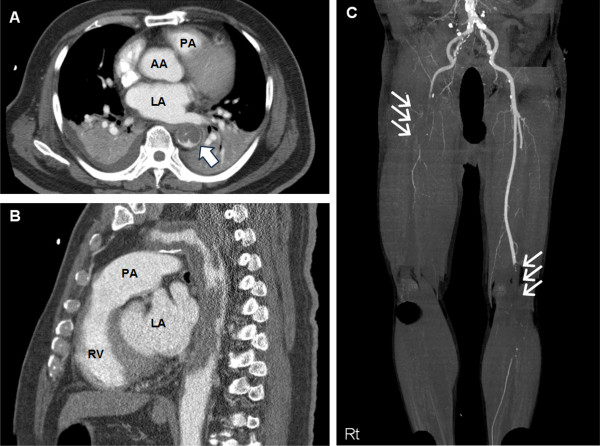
Images of the patient’s 3DCT before surgery. (A) Axial and (B) sagittal section 3DCT scans of the aorta show near total occlusion of the descending thoracic aorta that is blocked with diffusely distributed thrombus-like mass (single white arrow). (C) 3DCT scan of the lower extremities shows total occlusion of the right common iliac artery (right triple white arrows) and left superficial femoral artery (left triple white arrows). 3DCT: three-dimensional computed tomography; PA: pulmonary artery; AA: ascending aorta; LA: left atrium; RV: right ventricle.
